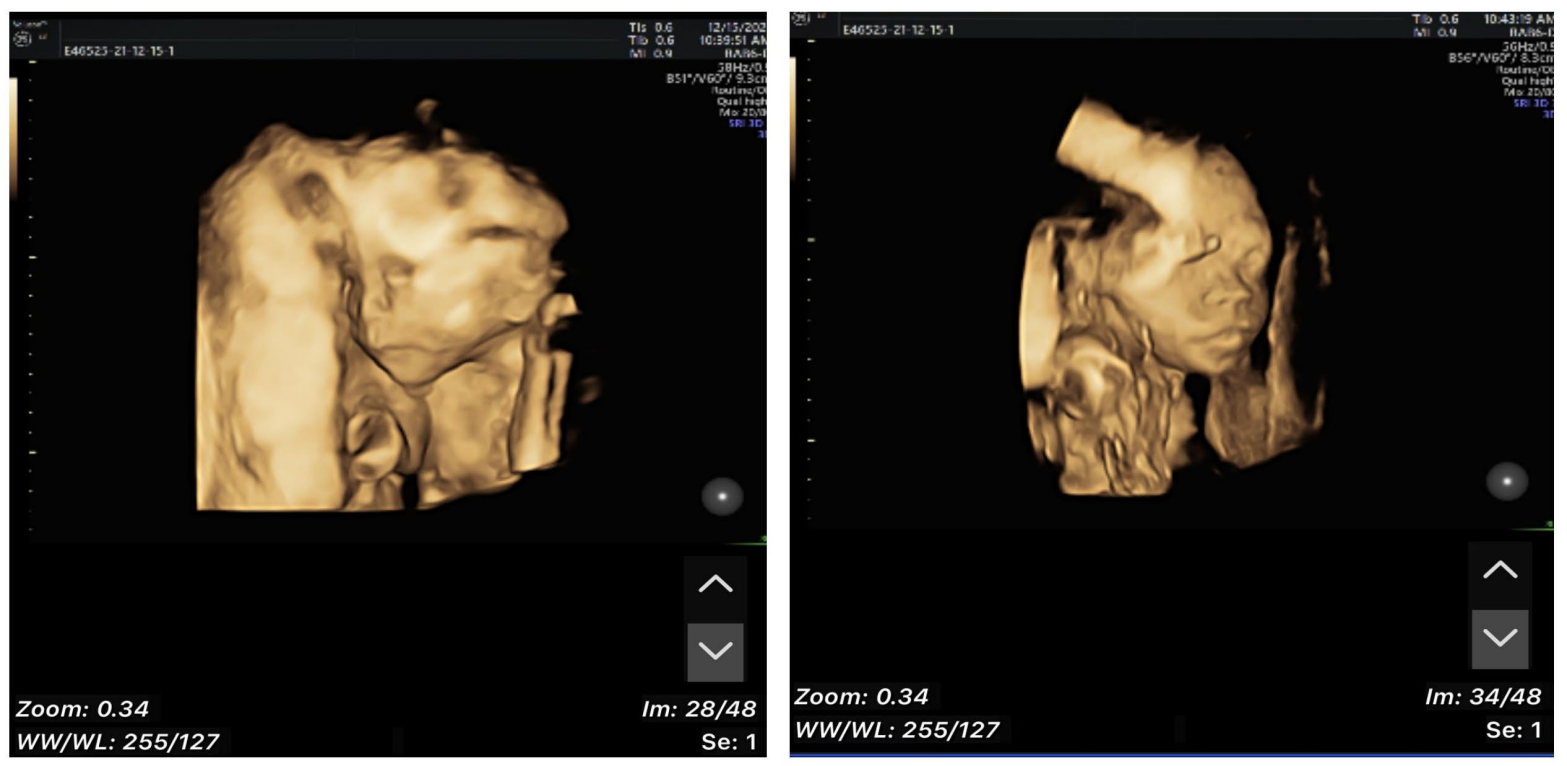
Fetal color ultrasound image at 21 weeks.
